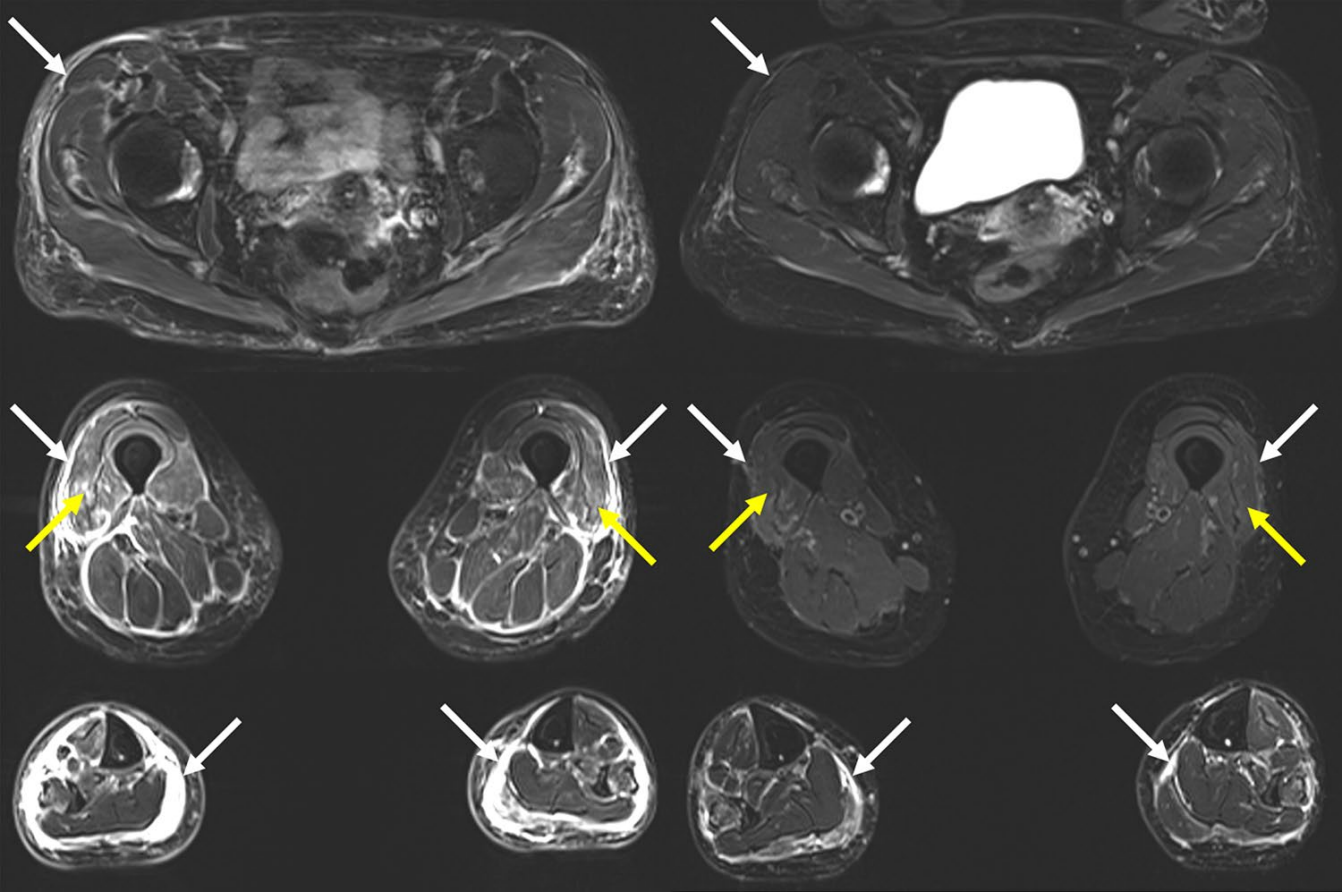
Muscle MRI, patient 2, at time of symptoms (left) and control after 5 months (right). Dixon “water” T2 weighted images, showing symmetrical (with slight right predominance) signs of myositis in vastus lateralis muscles (yellow arrows), and fasciitis (white arrows), predominant in the legs (yellow arrows)
